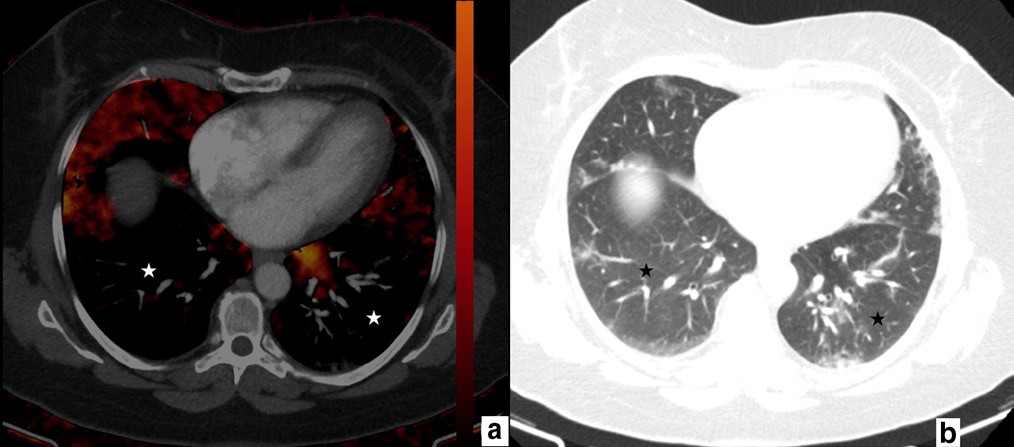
77-year-old male. Axial perfusion blood volume (a) and computed tomography (b) images. Peripherally distributed ground glass opacifications and focal consolidations are present (b), large areas of perfusion deficits in the bilateral lower lobes are present on perfusion blood volume images (a, stars). Perfusion deficit areas occupy a larger area than GGOs and consolidations and mainly appear normal on computed tomography (b, stars).
